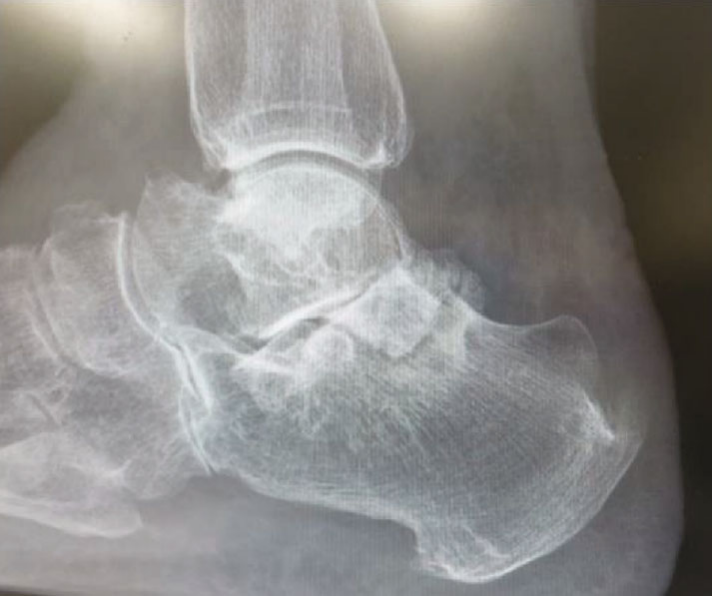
Lateral plain radiographic view of the right ankle showing no ossified loose bodies in the right posterior ankle extra-articular space.
